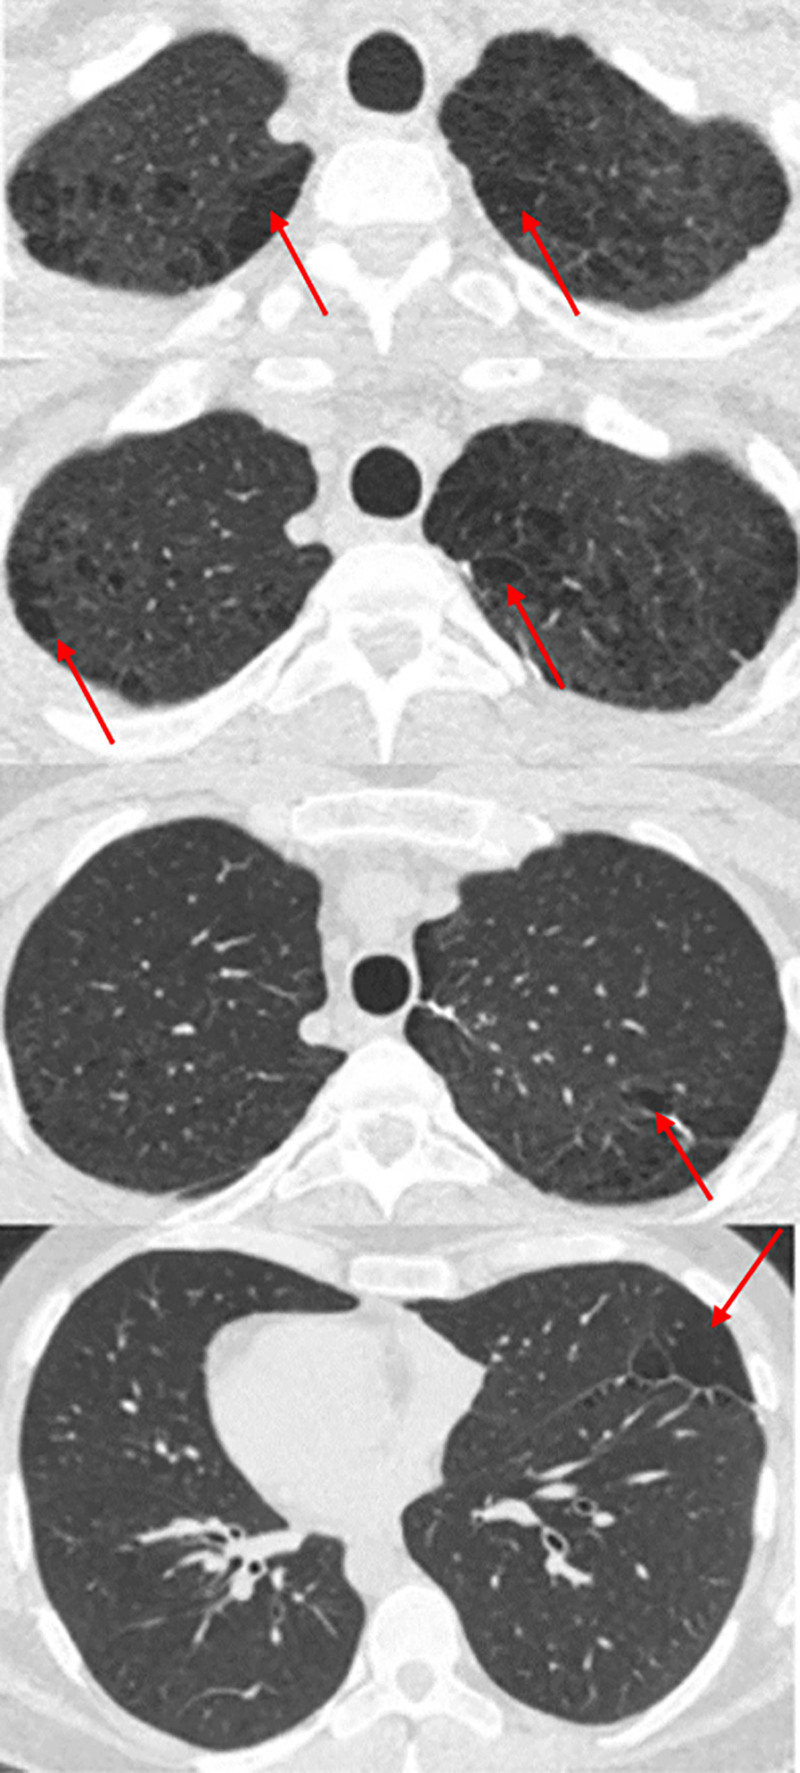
Thoracic computed tomography of the patient with secondary SP. SP = spontaneous pneumothorax.
